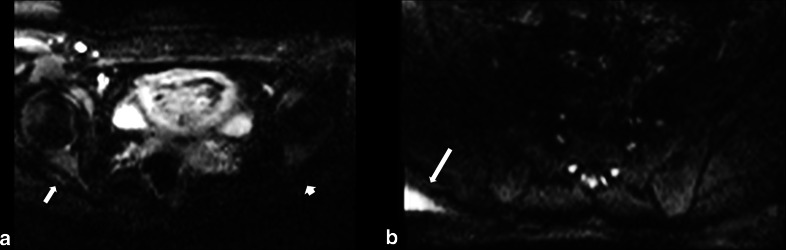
Demonstration of DWI-RESOLVE signal inhomogeneity. The b50 image (a) of a patient with normal neurophysiology testing demonstrates relatively prominent loss of signal on the left side of the body compared to the right. The left sciatic nerve (short arrow) is obscured due to this loss of signal. The normal right sciatic nerve (long arrow) appears asymmetric due to this spurious artifact. The b800 image (b) on a different patient demonstrates the hyperintense signal (arrow) that can occur at the edge of the field of view on some patients. This type of signal inhomogeneity does not typically obscure or distort the lumbosacral plexus
